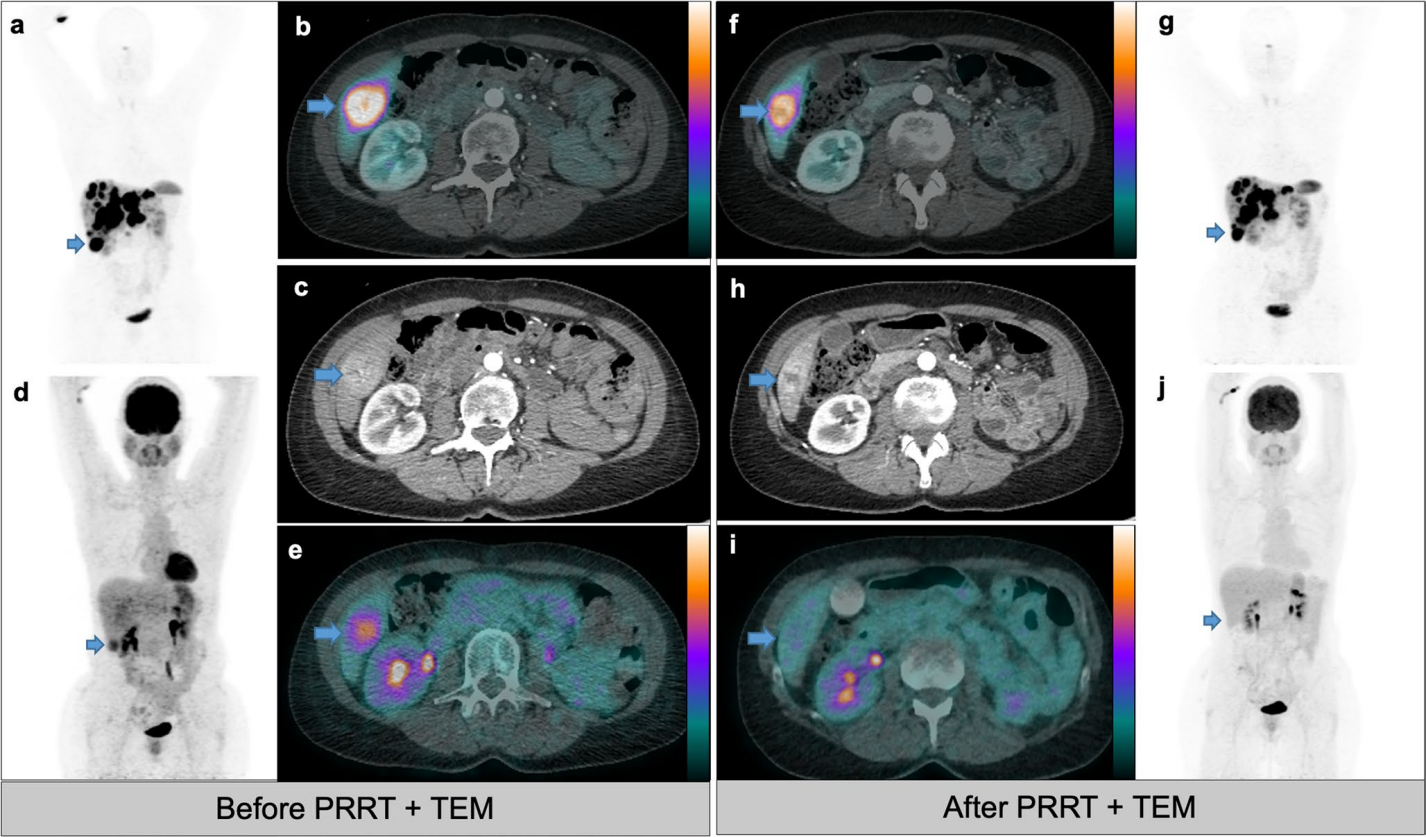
Patient treated with PRRT plus temozolomide (ID12). A 52-year-old female patient diagnosed in 2008 with gastric NET (G2, Ki67 8%). After progression under cold somatostatin analogs and previous PRRT, she presented diffuse liver metastases (a-e). The patient underwent a combination therapy of (rechallenge) peptide receptor radionuclide therapy (PRRT) plus temozolomide (TEM) 200 mg/m^2^/5d. Imaging performed after the end-of-treatment demonstrated stable disease on both [⁶⁸Ga]Ga -DOTATOC PET/CT (f,g) and ceCT (h) scans. Notably, the FDG-positive liver lesion in segment VI (d,e) exhibited a complete response after treatment (i,j), though a minor response was observed on [⁶⁸Ga]Ga-DOTATOC PET/CT and CT (blue arrows). The patient had a progression-free survival (PFS) of 25 months and an overall survival (OS) of 29 months
